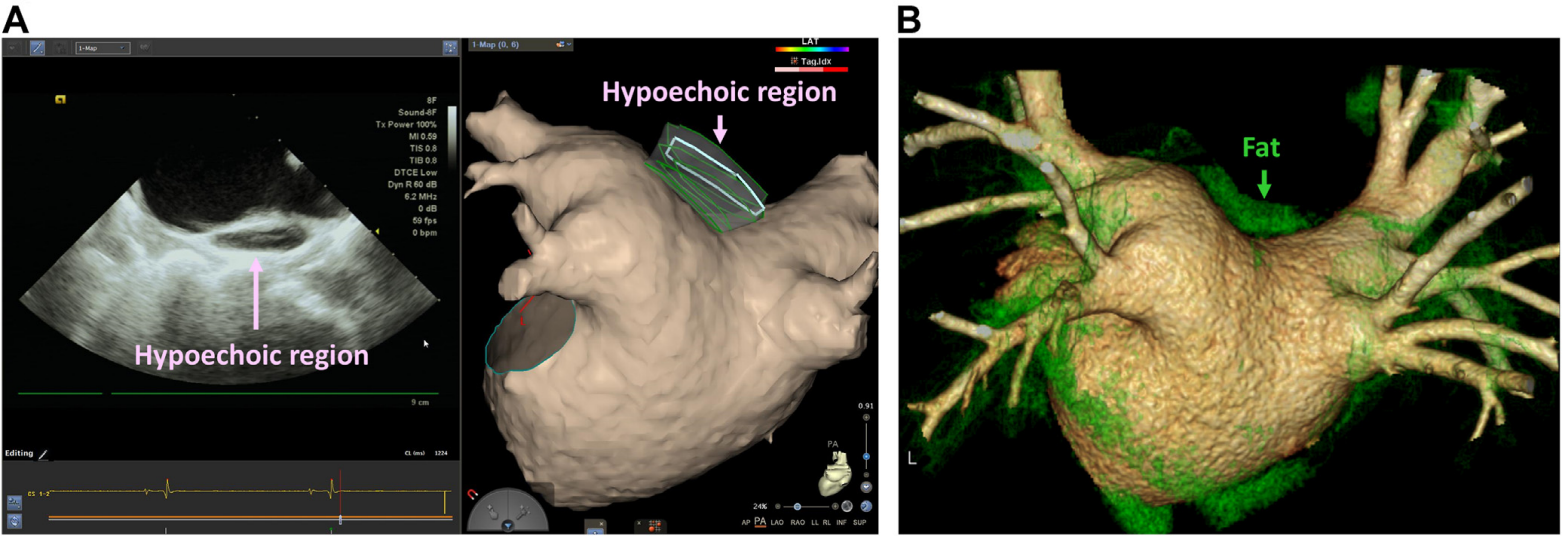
Validation with cardiac CT. A: ICE image showing a hypoechoic region on the LA roof. B: Cardiac CT image revealing fat around the LA. The fat region, with CT values of −50 to −100 Hounsfield unit, is highlighted in *green*. The hypoechoic region identified using ICE corresponds to the fat region reconstructed using cardiac CT. CT = computed tomography; ICE = intracardiac echocardiography; LA = left atrium/atrial.
